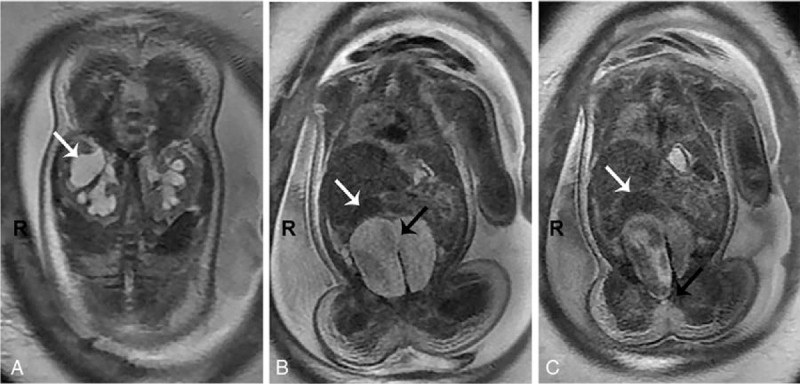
Prenatal MRI results. (A) The double collection system (white arrow) was found in the right kidney; (B) septum can be seen in the midline of the capsule (black arrow), but the upper part of the capsule is interconnected (white arrow); (C) the right cystic cavity passes down in the rectum and anal region (black arrow), and its upper end appears to be connected with the intestine (white arrow). MRI = magnetic resonance imaging.
